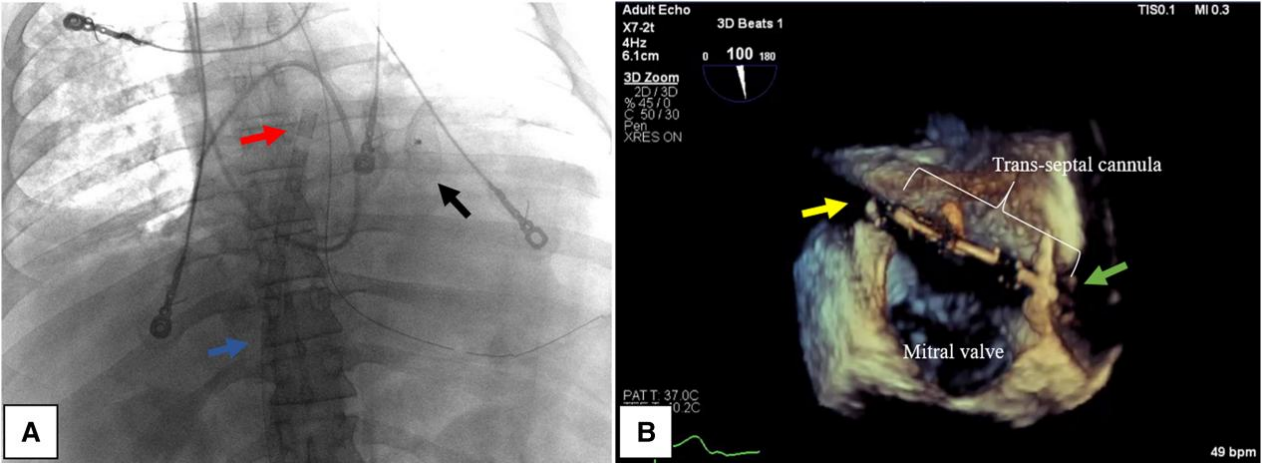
Imaging of the LAVA-ECMO cannulation in Patient 5. On X-ray imaging (*A*) demonstrating the proximal/right atrial (blue arrow) and distal/left atrial (red arrow) segments of the fenestrated cannula. A percutaneously deployed VSD closure device can be seen as well (black arrow). On TEE (*B*), the ECMO cannula can be visualized crossing the interatrial septum (green arrow) into the left atrium, with the distal end seated in the pulmonary vein (yellow arrow).
